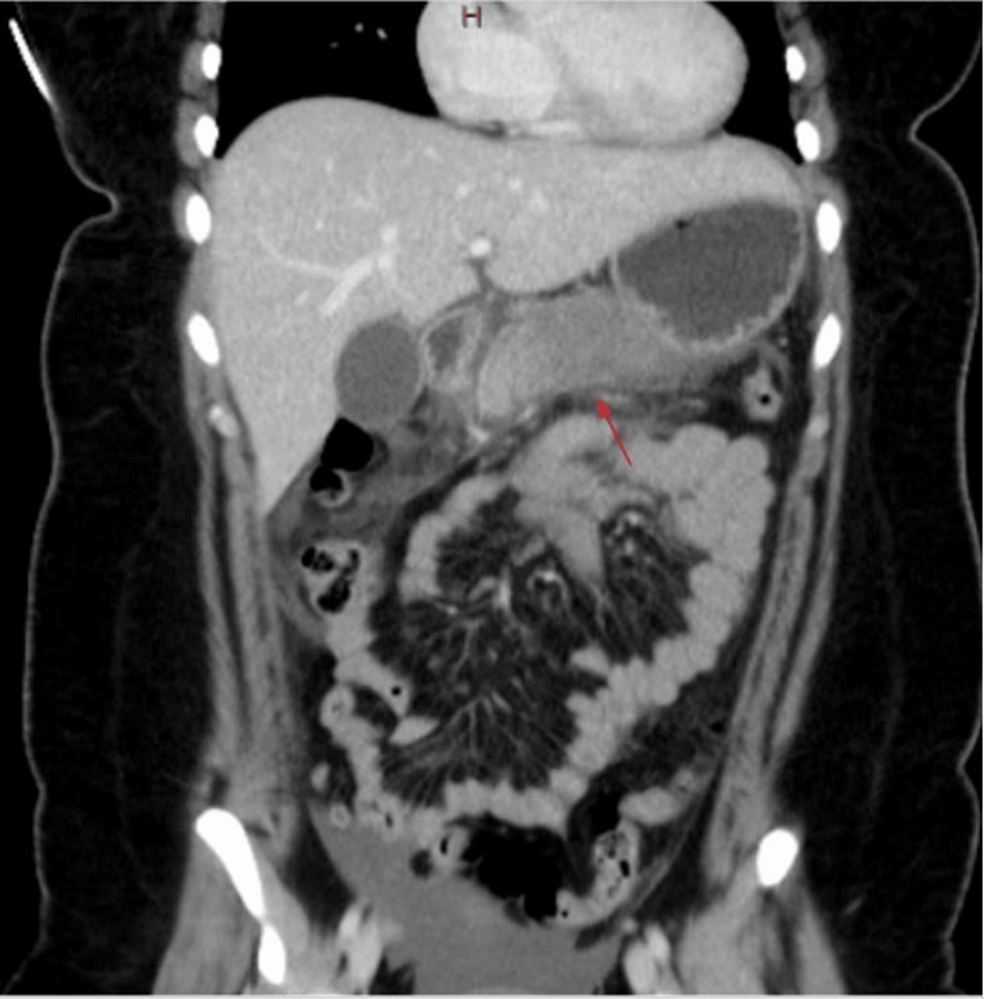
CT of the abdomen and pelvis with contrast The red arrow shows pancreatic edema
